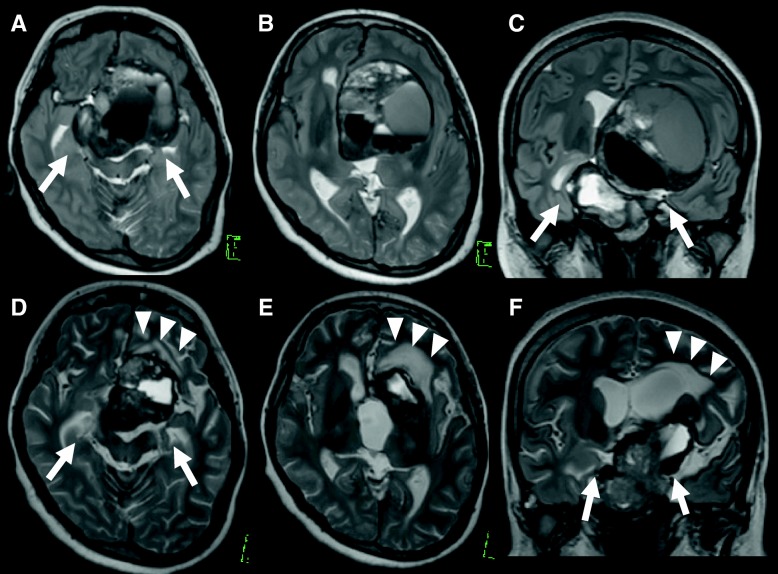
T2-weighted images (T2WI) before and after dopamine agonist therapy. a–c Before treatment. d–f After initial treatment. Following significant reduction of the tumor, the bilateral mesial temporal lobes returned to medial position (*white arrows*), and a hyperintense area on T2WI appeared in the left frontal lobe (*white arrowheads*)
